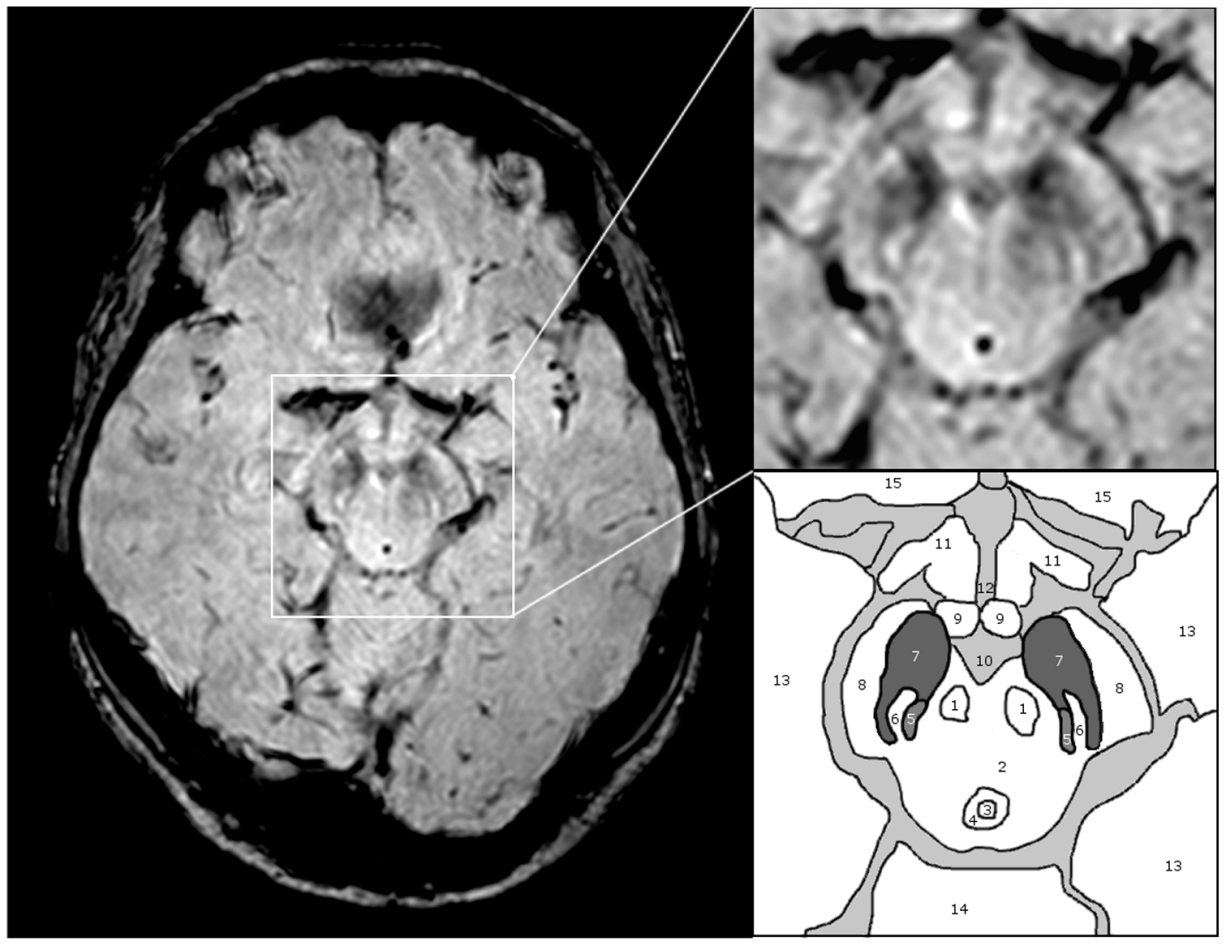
Substantia nigra anatomy on 3T - SWI – MRI. Demonstrated is a 3T - SWI axial slice just at the level of nigrosome-1 with magnification of the midbrain structures and a sketch outlining relevant anatomical structures: 1 red nucleus, 2 midbrain tegmentum, 3 aqueduct, 4 periaqueductal grey, 5 medial leminiscus, 6 nigrosome-1, 7 substantia nigra, 8 cerebral peduncle, 9 mammillary body, 10 inter-peduncular fossa, 11 optic radiation, 12 3^rd^ ventricle, 13 temporal lobe, 14 cerebellum, 15 frontal lobe.
